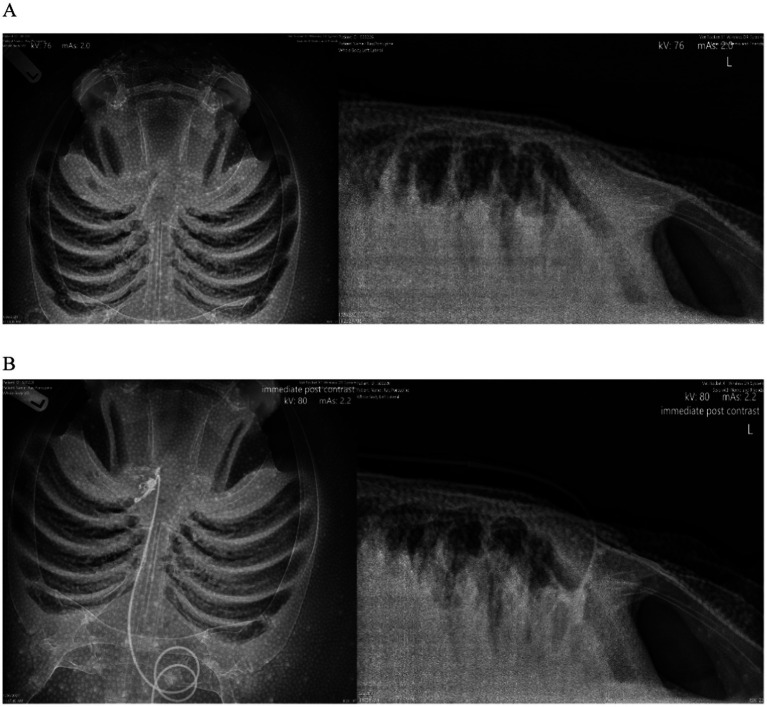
Pre and post contrast radiographic images of a porcupine ray (*Urogymnus asperrimus*) with endolymphatic system disease. (A) Survey view, ventrodorsal (VD) view and lateral. (B) Post iodinated-contrast infused into the affected area, VD view and lateral.
